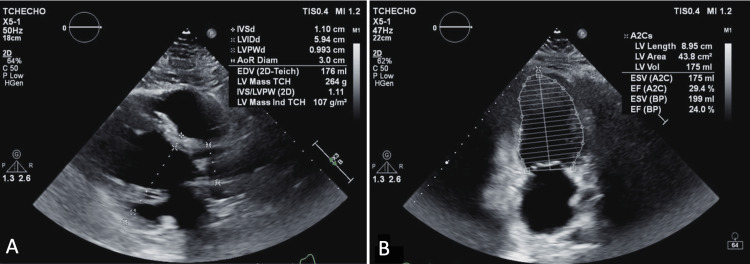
Parasternal long-axis (A) and two-chamber view (B) TTE demonstrated normal LV wall thickness and mass index. The LVEF is calculated at 24% by Simpson’s biplane method. TTE: transthoracic echocardiography; LV: left ventricular; LVEF: left ventricular ejection fraction
